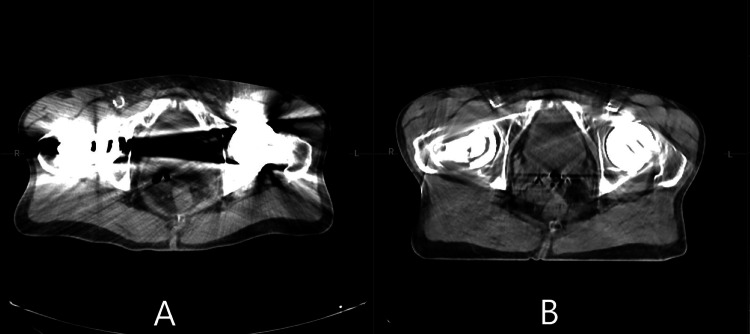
A) Planning CT image; B) HyperSight iCBCT with MAR iCBCT: Image-guided cone-beam computed tomography; MAR: Metal artifact reduction
